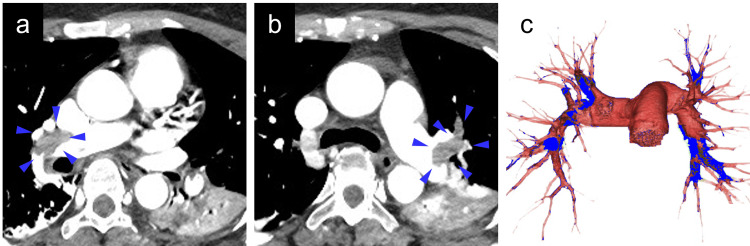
Contrast-enhanced CT (a, b) and 3D CT (c) showing massive PEs at eight days post-operation (a) PE in the segmental branches of the right pulmonary arteries (blue arrowheads). (b) PE in the main and segmental branches of the left pulmonary artery (blue arrowheads). (c) Multiple bilateral PEs (blue area) in the pulmonary artery (red area). PE, pulmonary embolism
